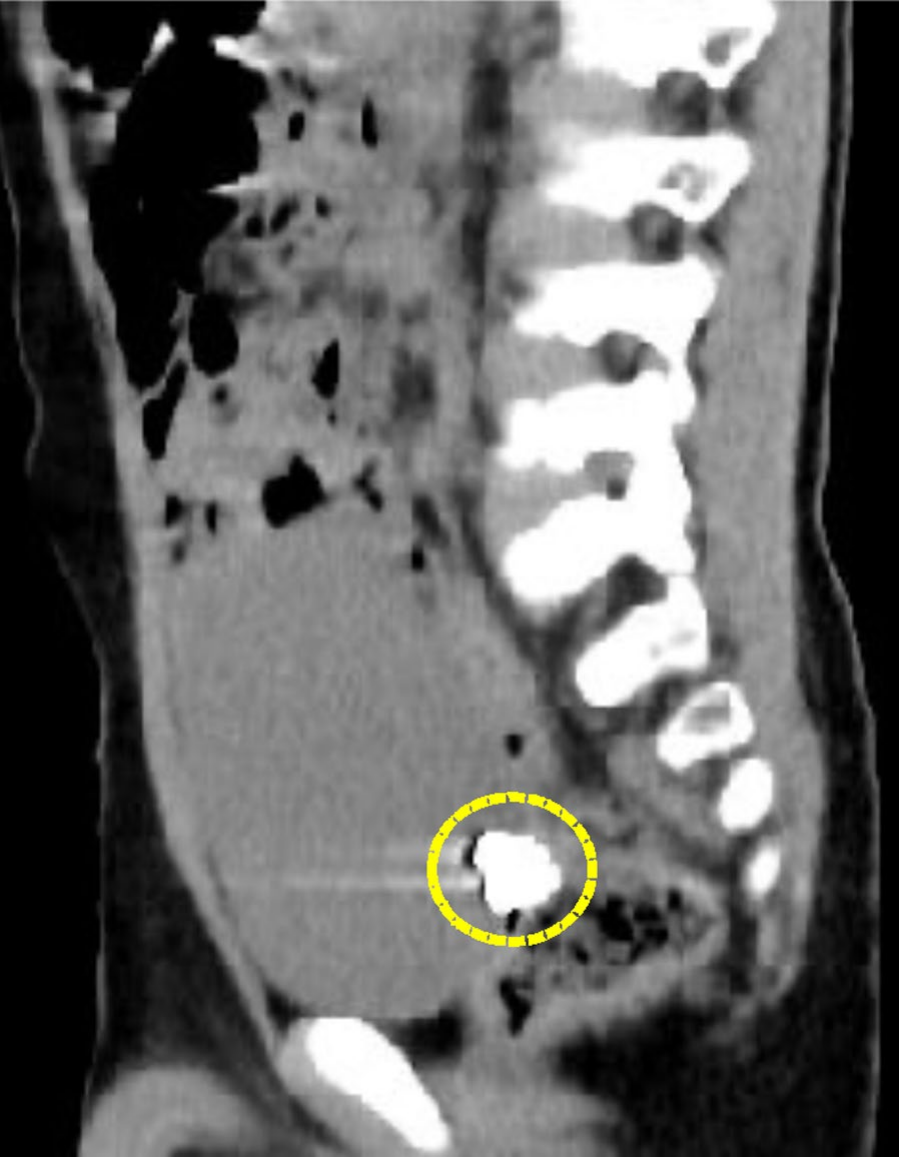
CT imaging (sagittal). On CT, the FB (yellow circle) was suspected to locate in the terminal ileum dorsal side to the bladder. There were no characteristic findings of SH that the bladder was suppressed by the incarcerated small bowel
